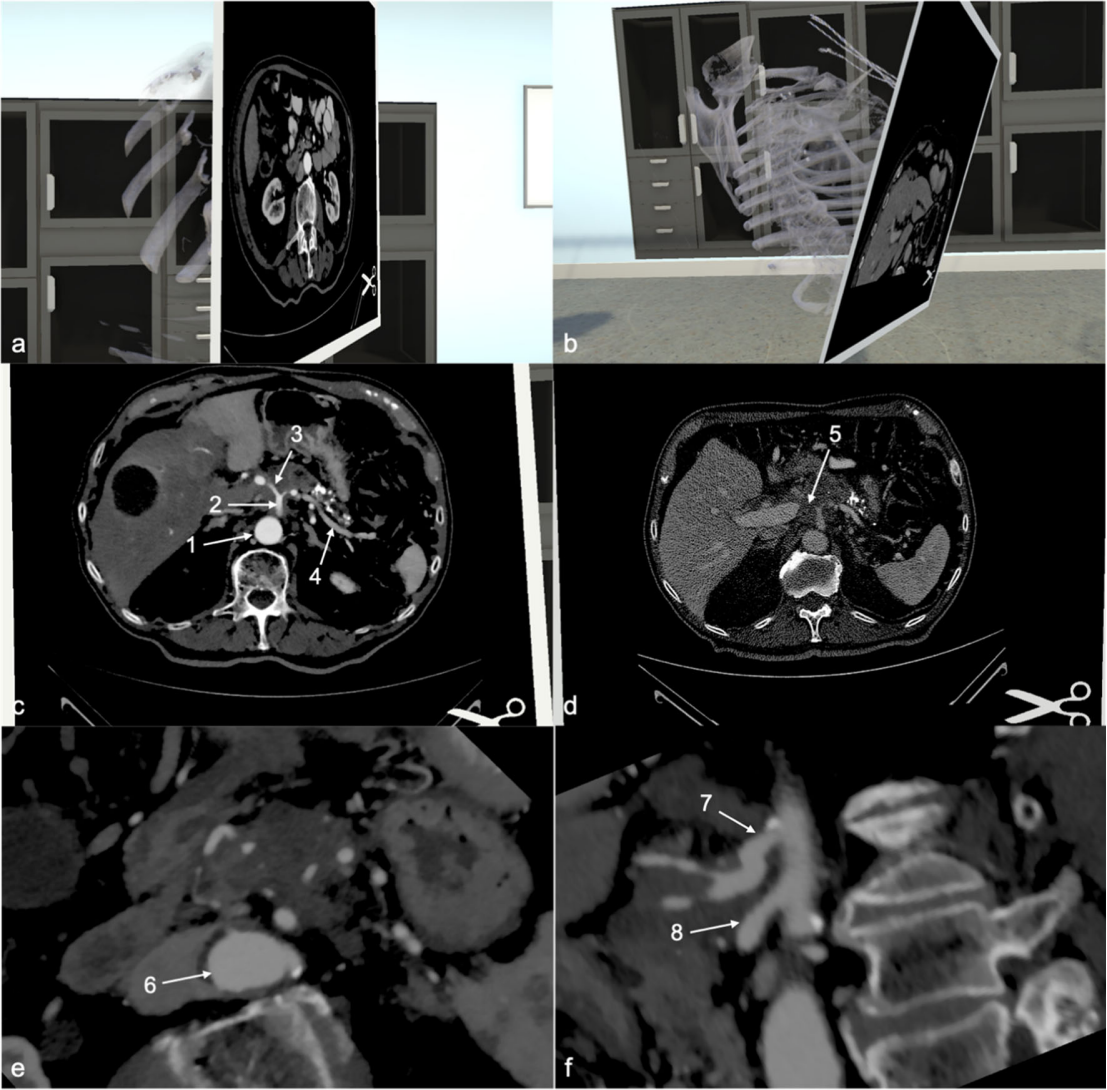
CT model of locally advanced pancreatic cancer. a Section through the CT model of a patient with LA pancreatic cancer. b The cutting plane can be placed in any desired angle. c Overview of an axial section: (1) Aorta (2) Celiac trunk (3) Common hepatic artery (4) Splenic artery. d Light–Dark contrast can be adapted for each model. The tumour is clearly visible on this section (5). e, f Tumour encasement of vessels is seen in various sections from different angles: (6) Aorta (7) Celiac trunk (8) Superior mesenteric artery
